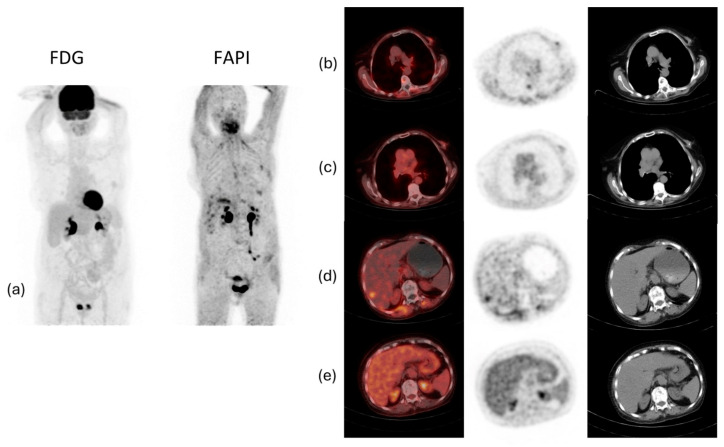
^18^F-FDG and ^68^Ga-fibroblast activation protein inhibitor-46 (^68^Ga-FAPI-46) PET/CT images of a 74-year-old woman with invasive lobular carcinoma of the left breast. (a) Maximum intensity projection (MIP) whole-body PET/CT images. (b) ^68^Ga-FAPI-46 PET/CT of the primary breast lesion. (c) ^18^F-FDG PET/CT of the primary breast lesion. (d) ^68^Ga-FAPI-46 PET/CT of the metastatic hepatic lesions. (e) ^18^F-FDG PET/CT of the metastatic hepatic lesions. The images show faint ^18^F-FDG uptake in the left breast, increased ^68^Ga-FAPI uptake in the upper central quadrant of the left breast, ^68^Ga-FAPI-46 avid metastatic hepatic lesions, and ^68^Ga-FAPI-46 avid pleural thickening in the right lower lobe of the lung (likely atelectatic).
